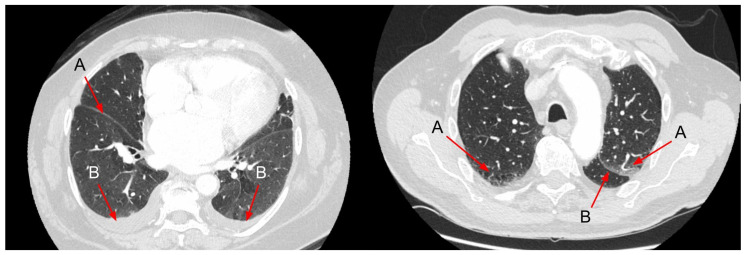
(left) Image of the lungs in CT in a patient with HF. Note the non-homogenous increased diffuse parenchymal density of the lungs. A: Thickening of interlobar fissure; B: Bilateral pleural effusion. (right) A: Cephalisation—widened upper lobe vasculature. B: Thickening of interlobar fissure and marginally increased density of the interstitium with a patchy appearance in the gravity-dependent part of the lungs within the lobes. Figure obtained with permission from the 2nd Department of Radiology, Medical University of Warsaw.
